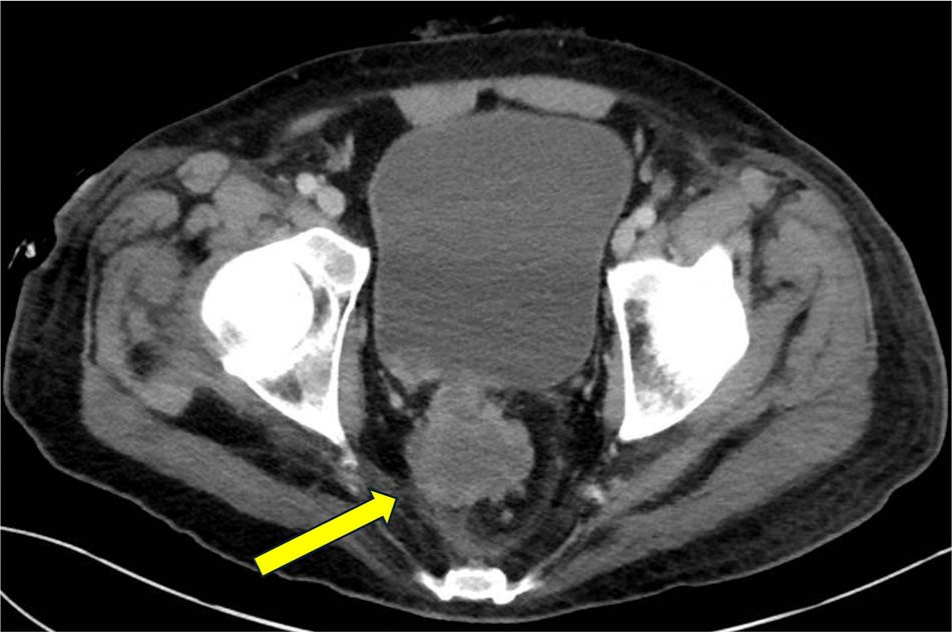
CT abdomen and pelvis—coronal plane. Primary rectal tumor measuring 4.3 × 4.5 cm.
